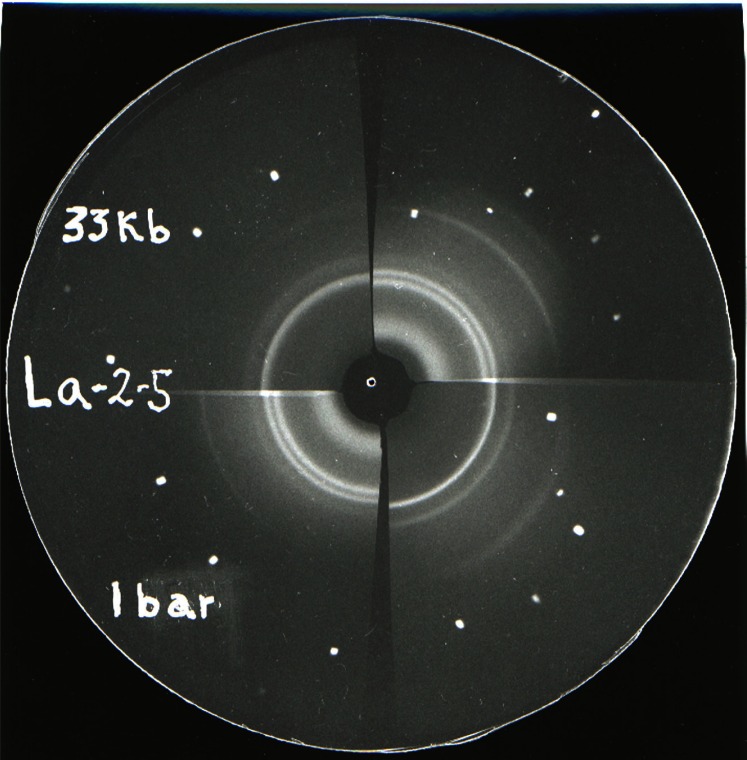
Matched pair (opposed quadrants) of x-ray powder patterns of the rare-earth element, La, taken at ambient pressure and 3.3 GPa (33 kbar). The hexagonal structure, stable at ambient pressure, transforms to a cubic-closed-packed structure at about 3.3 GPa.
